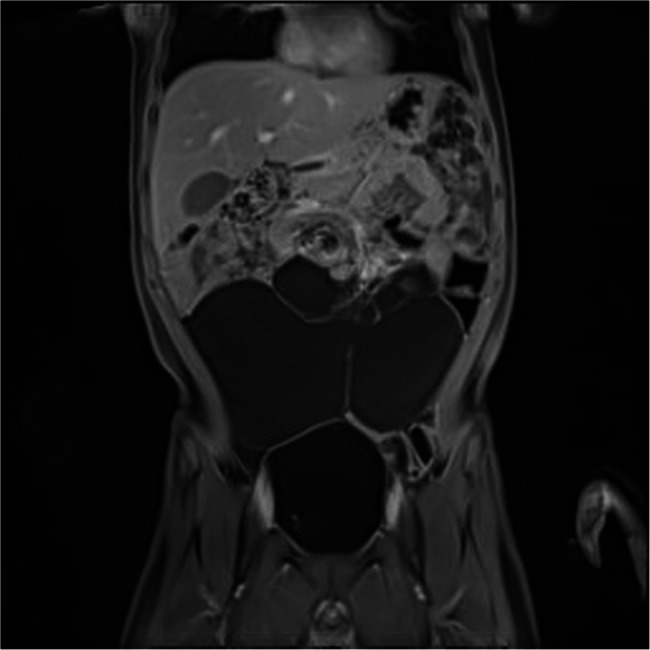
MRI image of abdominal lymphangioma
